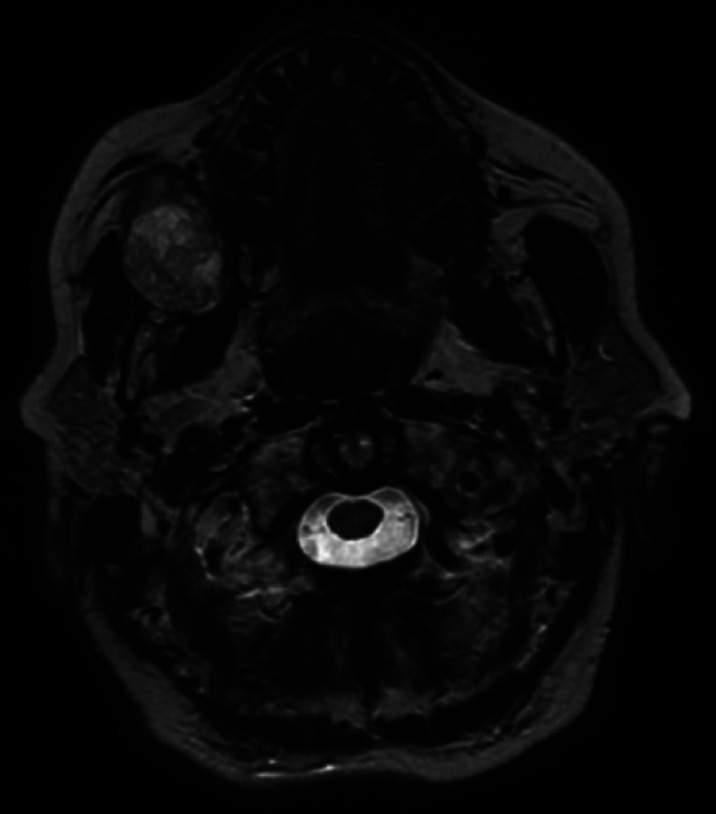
MRI. Axial T2-weighted images showing a mass with well-defined margins, smooth borders, slight inhomogeneous pattern and high-intensity signal; the mass seems encapsulated.
